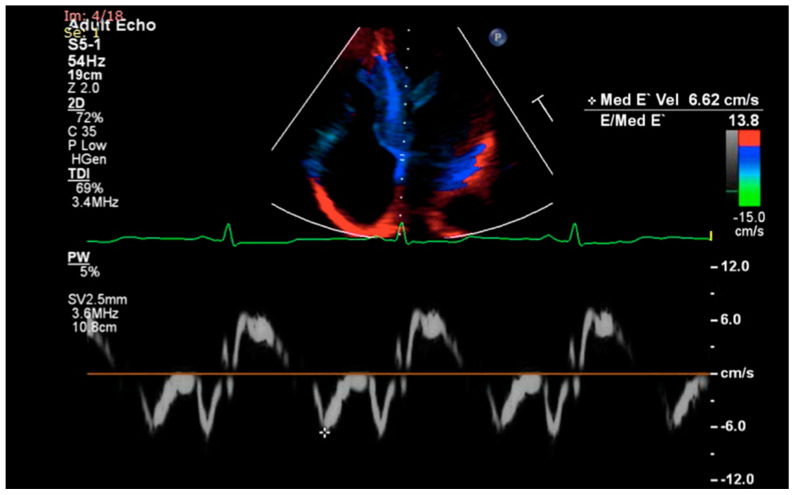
Tissue Doppler imaging demonstrating decreased septal e’ velocity and increased E/e’ ratio in a female with cardiac amyloidosis.
